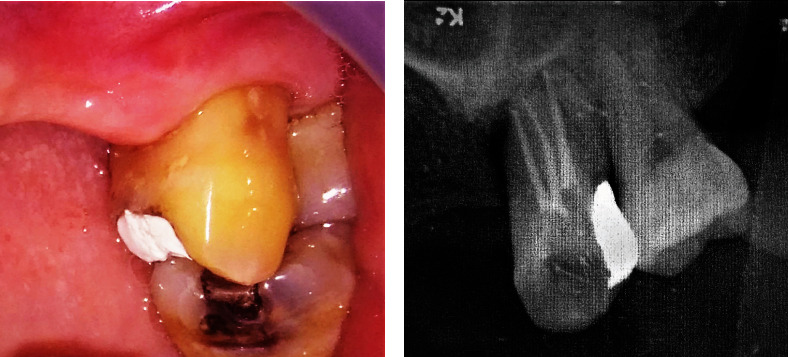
Clinical and radiographic examination of tooth #26 after root canal treatment. (a) Temporary filling covered the mesio-occlusal part of crown tooth #26. Gingiva looked normal. (b) Radiographic examination showed obturation of root canals of tooth #26 with decreased radiolucency on the apical part of the tooth.
